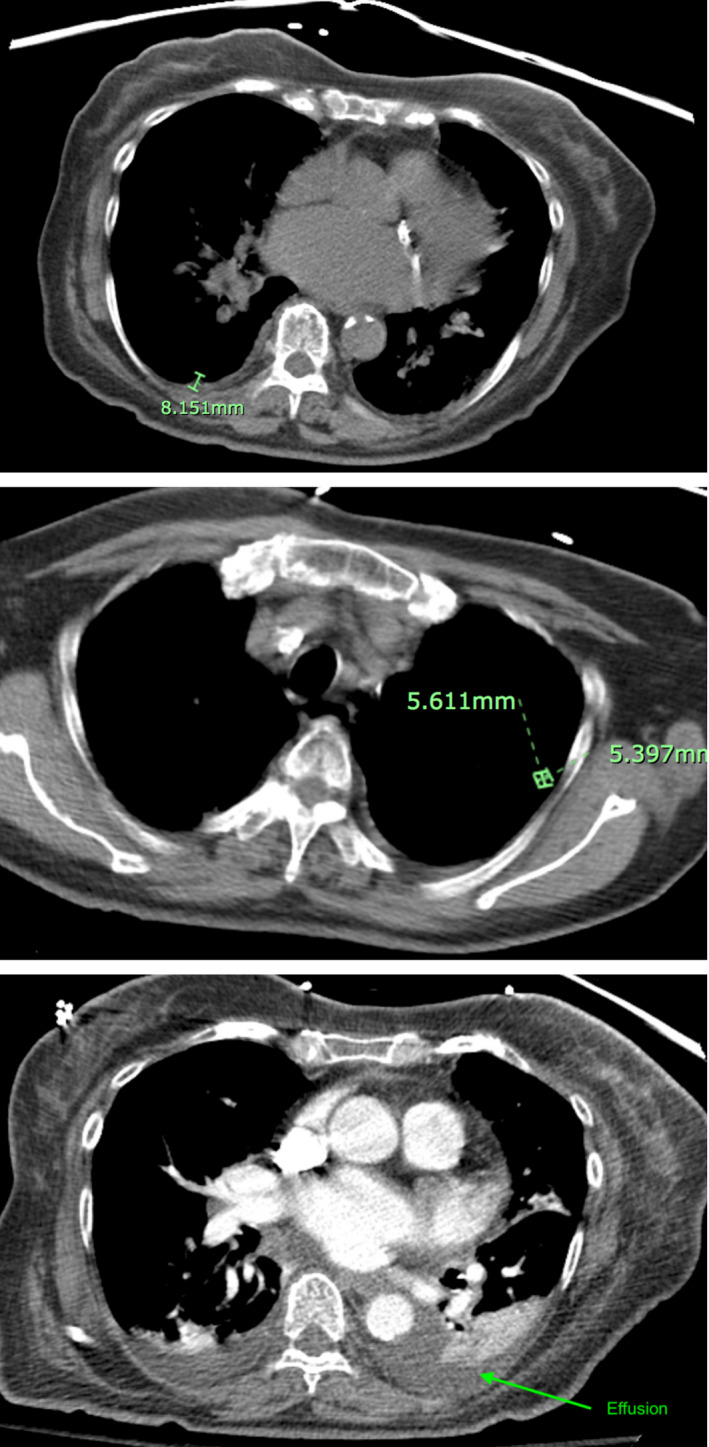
Chest CT scan without contrast showing 8.2 mm pleural nodule in right lung base (top), a 5.4 × 5.6 mm left upper lobe nodule (middle) and left lower lobe pleural effusion (bottom) that all together are suggestive of neoplasm
